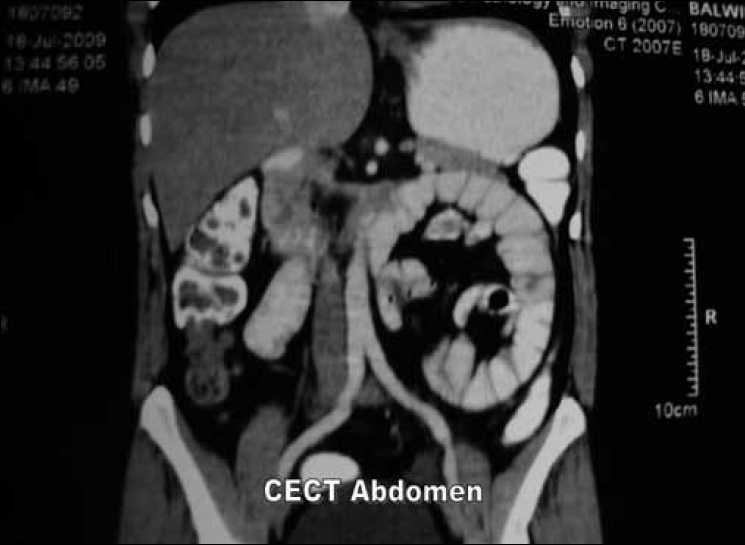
Contrast-enhanced CT scan of abdomen (CECT).
